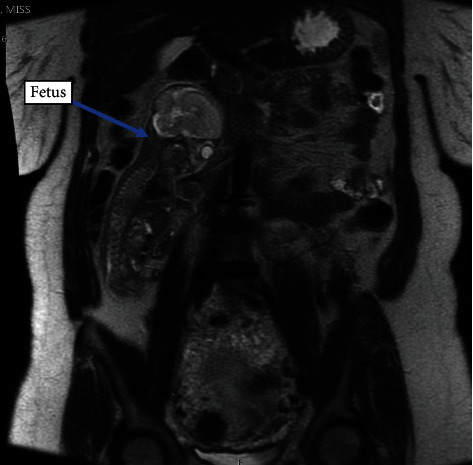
Abdominal MRI showing the fetus outside the uterus on the right side of the abdomen with the fetal head adjacent to the liver and gallbladder. The placenta is seen outside the uterus in the abdomen superior to the uterus.
